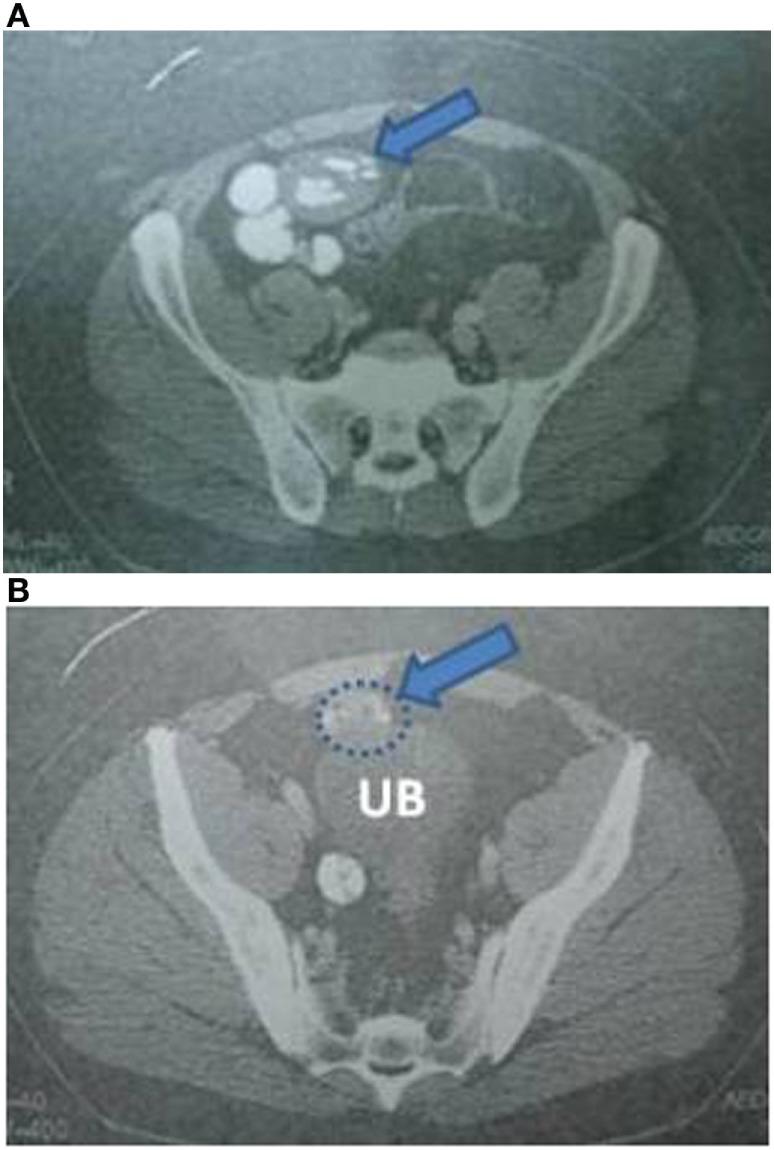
(A) Abdominal CT indicating an intra-abdominal cystic lesion, located near an ileal loop. (B) Abdominal CT indicating the aforementioned cystic lesion causing external pressure to the urinary bladder, and a smaller cyst at the right side of the wall of the urinary bladder.
